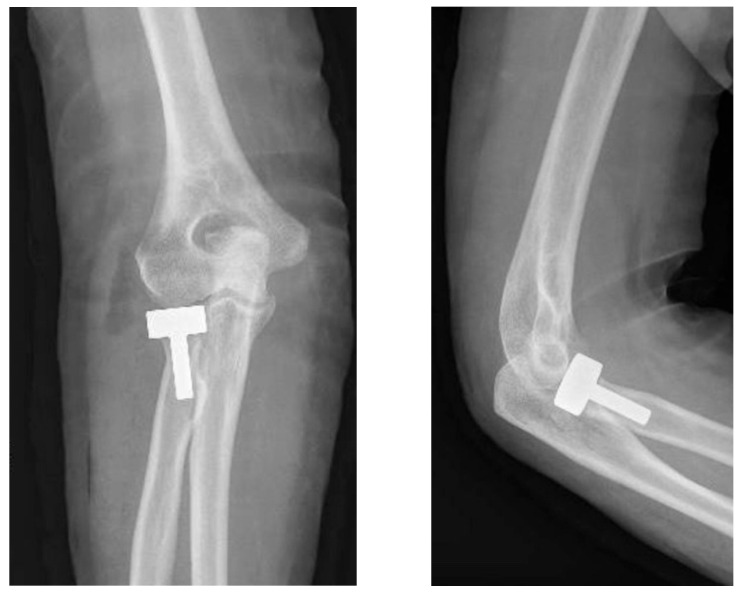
Radiographical AP and LL view of the implanted loose-fit monobloc radial head prosthesis.
